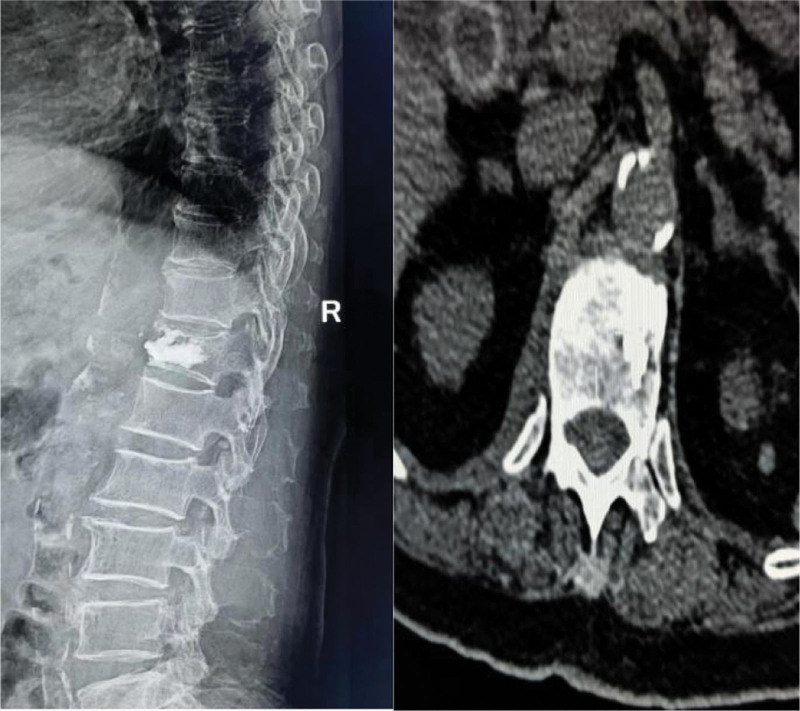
In a 73-year-old woman, we performed PKP for her T12 fracture. Postoperative follow-up DR and CT showed leakage of paravertebral bone cement. However, the patient did not have any symptoms. PKP = percutaneous kyphoplasty.
